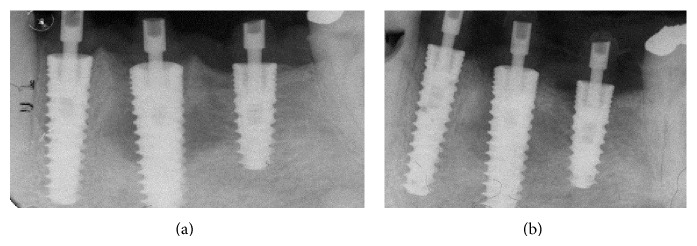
X-ray showing an optimum crestal and mesial distal position of the implants.
